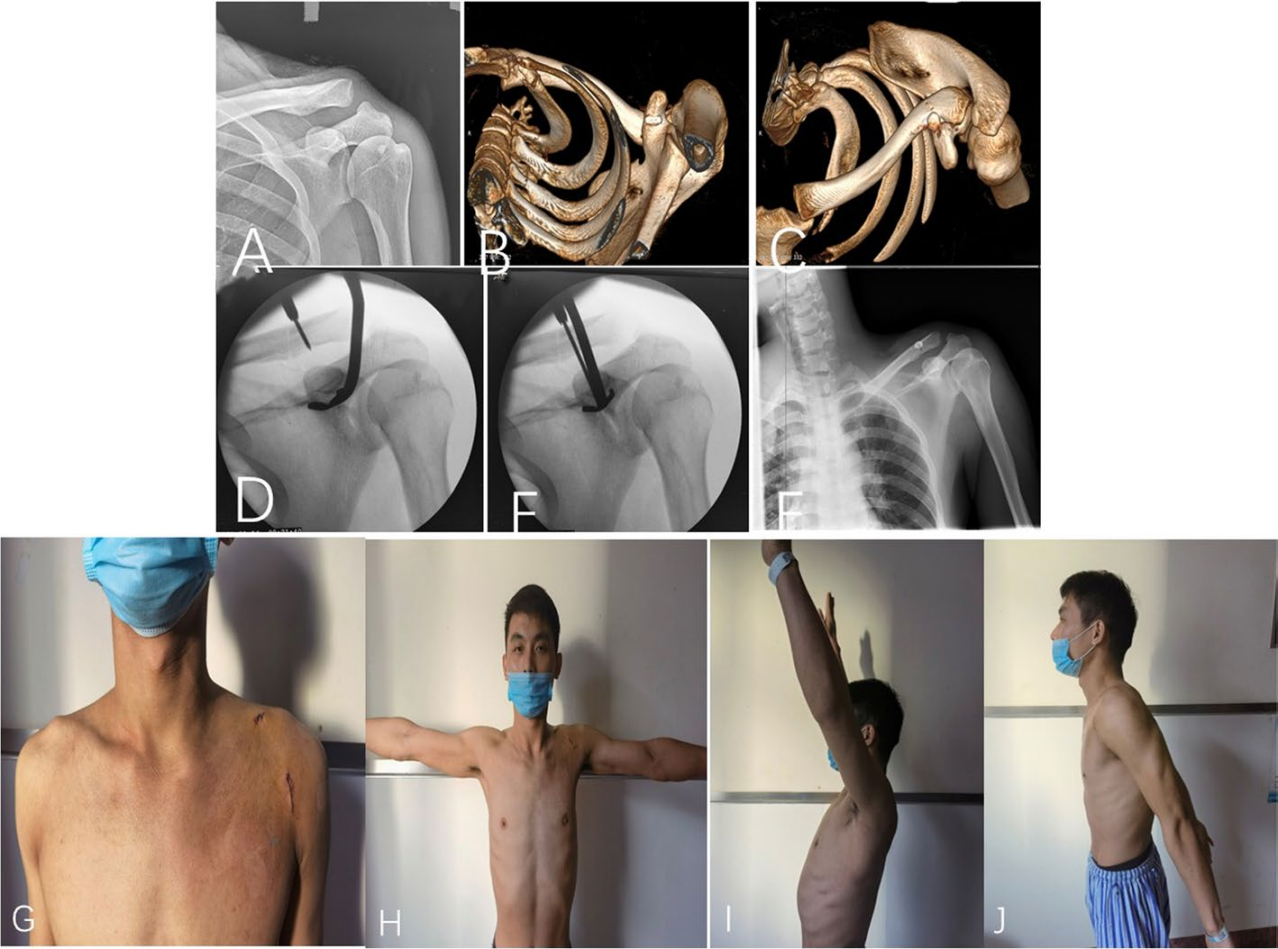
A-F Depict the operative procedure process of MTRLPF in a 29-year male patient, who had Rockwood type III acromioclavicular joint dislocation at his left shoulder due to the fall from standing height. A-C presents the preoperative radiograph (A) and three-dimensional reconstruction for the acromioclavicular joint dislocation. D-E presents the intraoperative operation and F shows the acceptable reduction. G-J depict the postoperative shoulder motion range at the 2^nd^ day after operation, showing almost complete recovery
